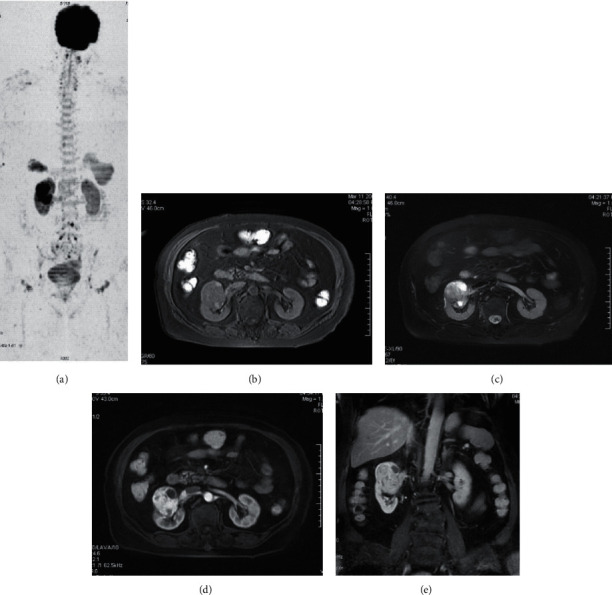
Kidney cancer. (a) WB-DWI image showed a significant low signal shadow in the right kidney with a range of 5.3 cm × 4.4 cm. (b) T1WI showed the lesion with low signal. (c) Lipid-suppressed T2WI showed hyperintense lesions with well-defined edges and areas of liquefaction necrosis. (d) Gd-DTPA dynamic enhanced scanning in arterial phase, the solid part of the lesion was significantly enhanced with clear boundary. (e) Lesion enhancement decreased during the coronal equilibrium phase.
